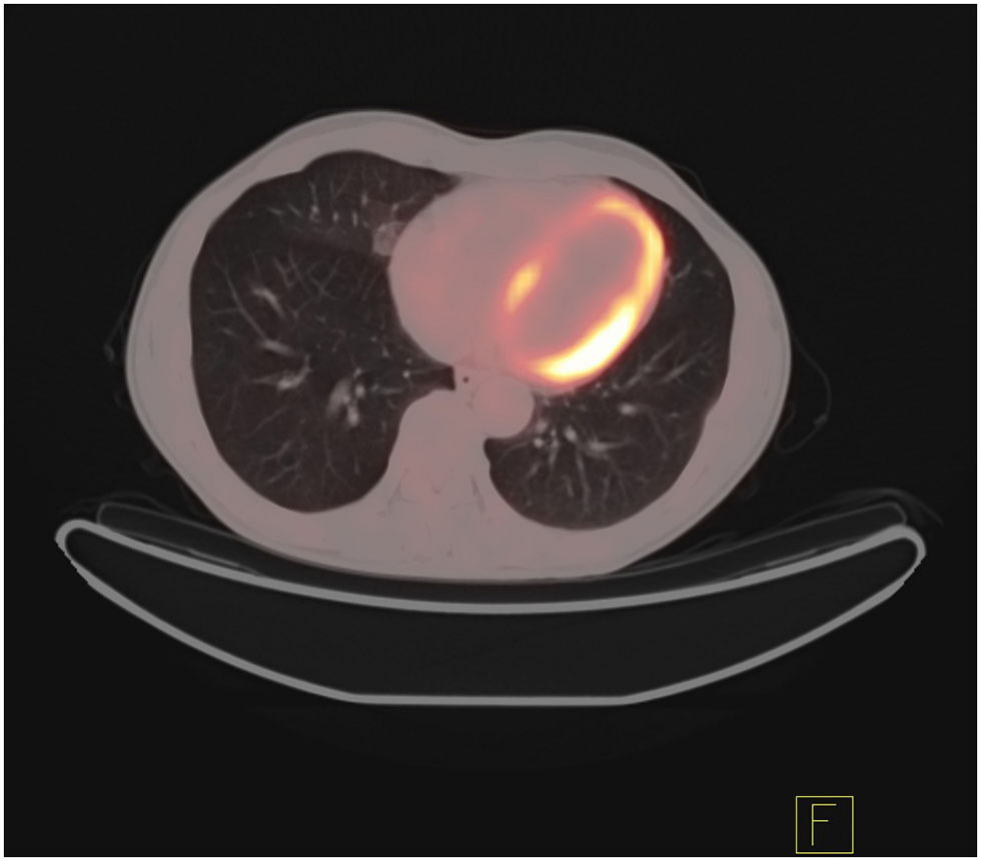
Non-metabolized granulomatous inflammation on PET/CT.
